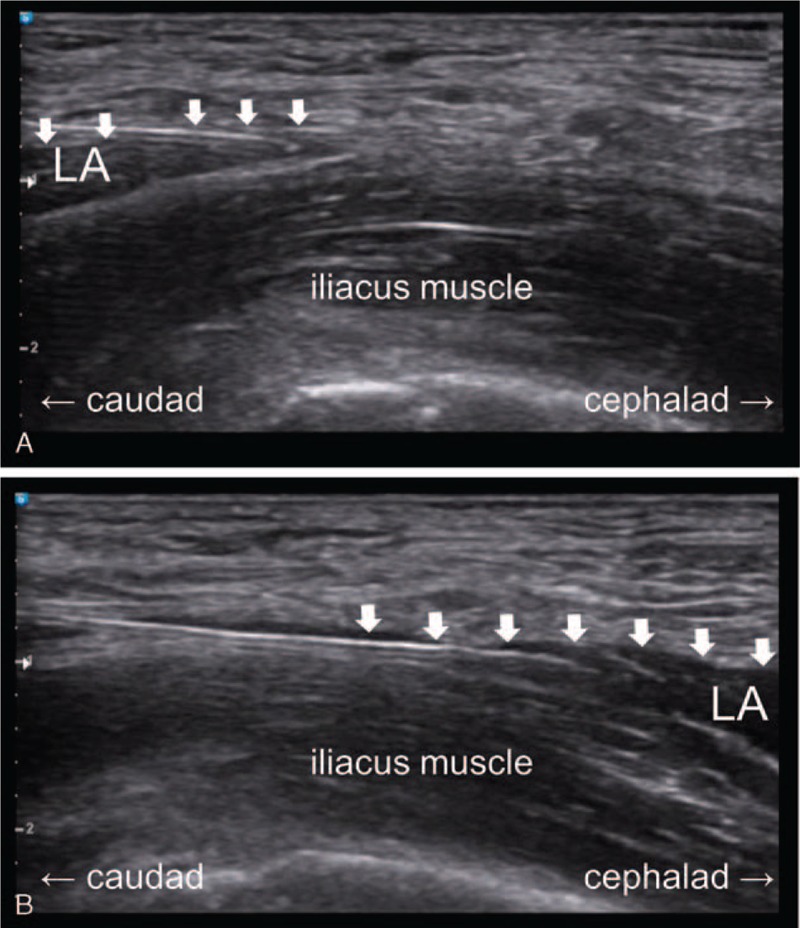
The heel-toe maneuver for needle visualization, when the needle is advanced. The needle is inserted through the skin using an in plane technique under ultrasonographic guidance. After confirming the passage of the needle through the fascia iliaca, a small amount normal saline is injected between the fascia iliaca and iliacus muscle. Longitudinal parasagittal view of the needle with its tip in position under fascia iliaca (A). Cephalad spread of the local anesthetic beneath the facia iliaca visualized in real time (B). For proximal spreading of local anesthetic, a small amount of solution is injected, followed by advancing the needle tip proximally using hydrodissection technique under ultrasonographic guidance, repeatedly. The fascia iliaca is indicated by line arrows. LA = local anesthetic.
